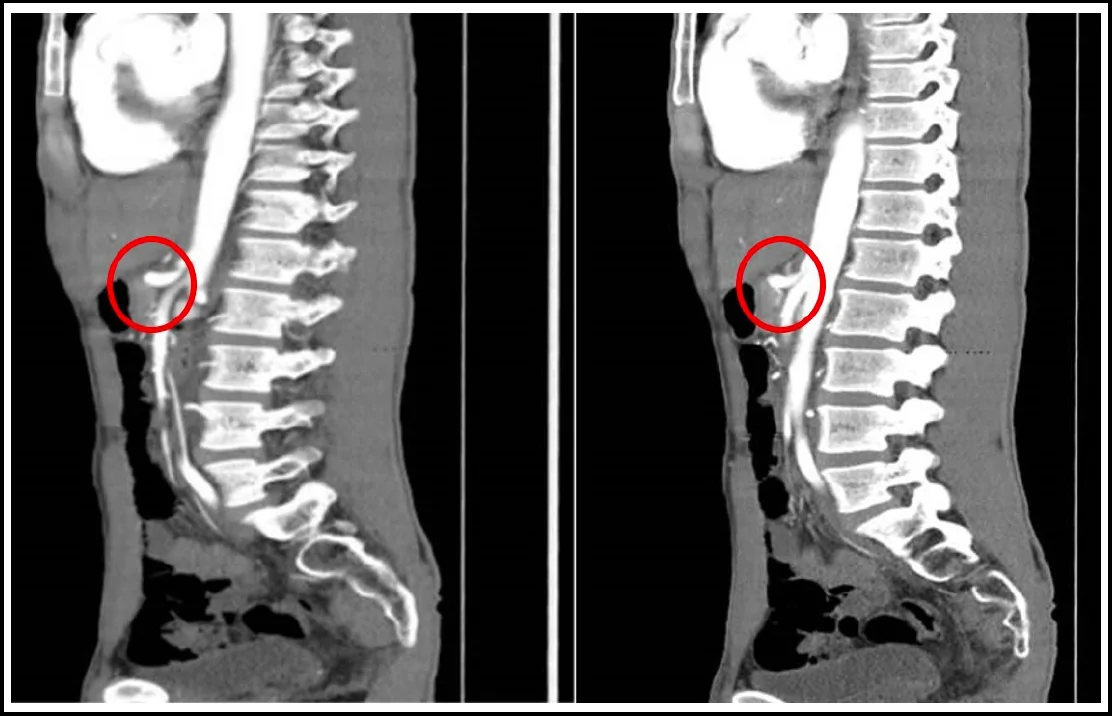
Computed Tomography Scan.
